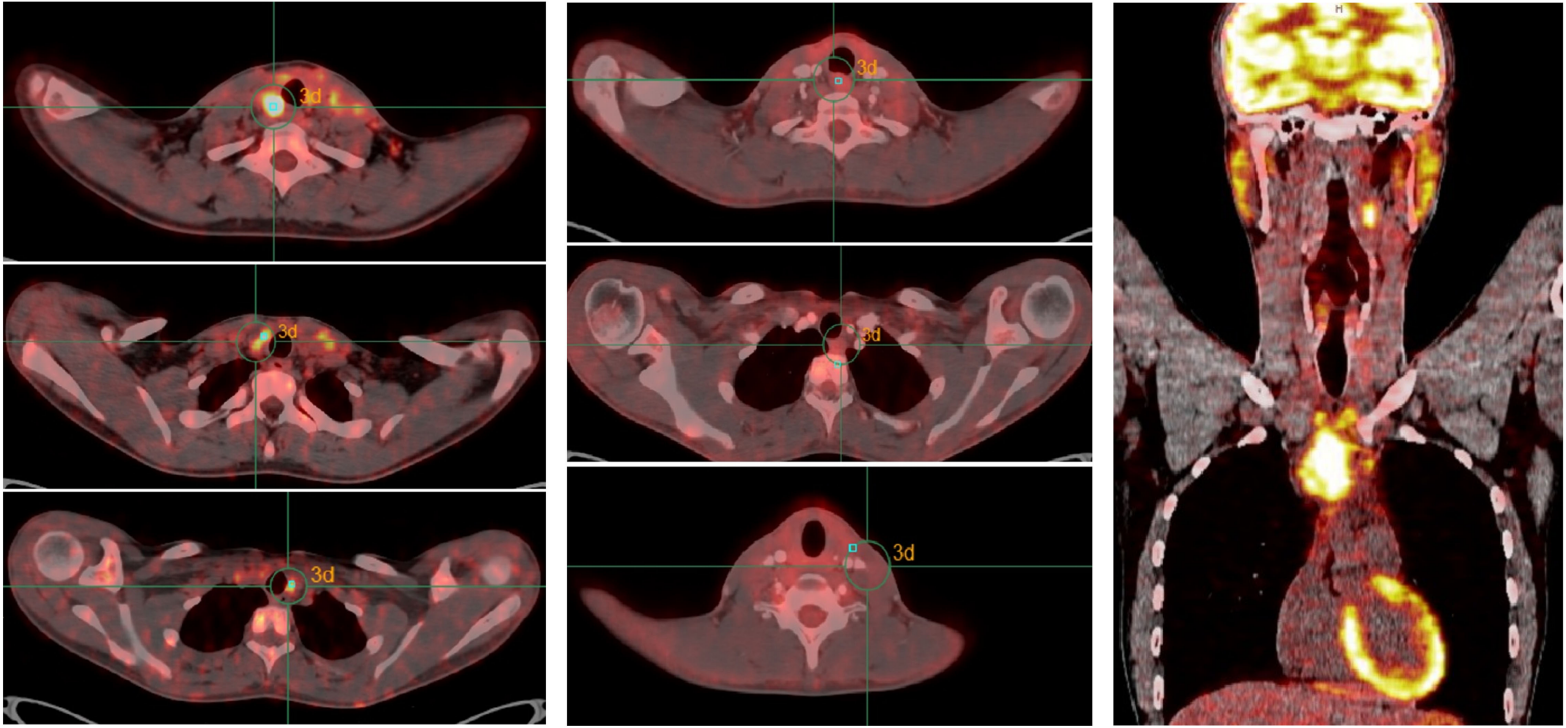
Radiological evolution under first-line treatment with ceritinib. (Left) Before treatment started, PET/CT scan shows signs of bilateral laterocervical adenopathy, as well as central compartment, and left supraclavicular lymph nodes. (Middle) Alter six cycles, normalization or very marked improvement of metabolic activity is observed in all previously detected laterocervical, supraclavicular and paratracheal lymph nodes. (Right) At progression, PET-CT scan showing evidence of local recurrence at the level of the left surgical bed and extensive infiltrative adenopathic involvement in the anterior mediastinum, as well as at level II left laterocervical and homolateral supraclavicular. No evidence of lesions suggestive of pulmonary or bone metastases.
